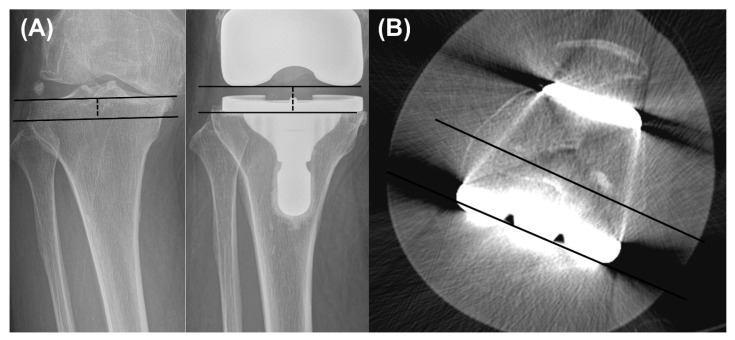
Measurement of (A) joint line distance on anteroposterior radiographs and (B) femoral component rotation angle on CT scan axial view.
